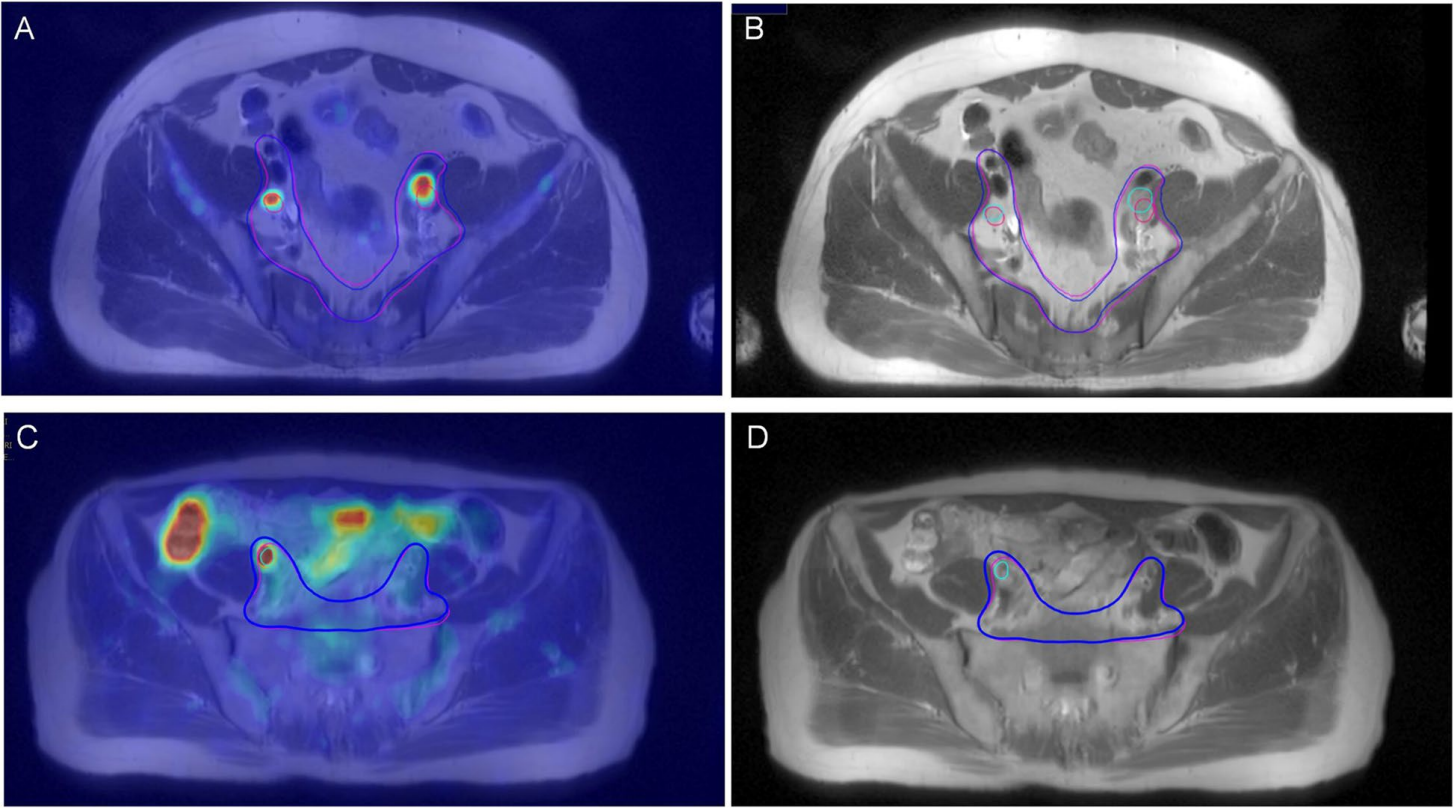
Target volume delineation for a 68-year-old man with high-risk PCa who was assumed two lymph node metastases based on PET/MRI (T2-weighted), leading to two GTVs (green line), resulting CTV in blue line (A). Target volume delineation based on MRI (T2-weighted) assuming two lymph node metastases leading to two GTVs (pink line), resulting CTV in pink line (B). Target volume delineation for a 67-year-old man with high-risk PCa who was assumed one lymph node metastasis based on PET/MRI (T2-weighted), leading to one GTV (green line), resulting CTV in blue line (C). Target volume delineation based on MRI (T2-weighted) assuming no lymph node metastasis, resulting CTV in pink line (D)
